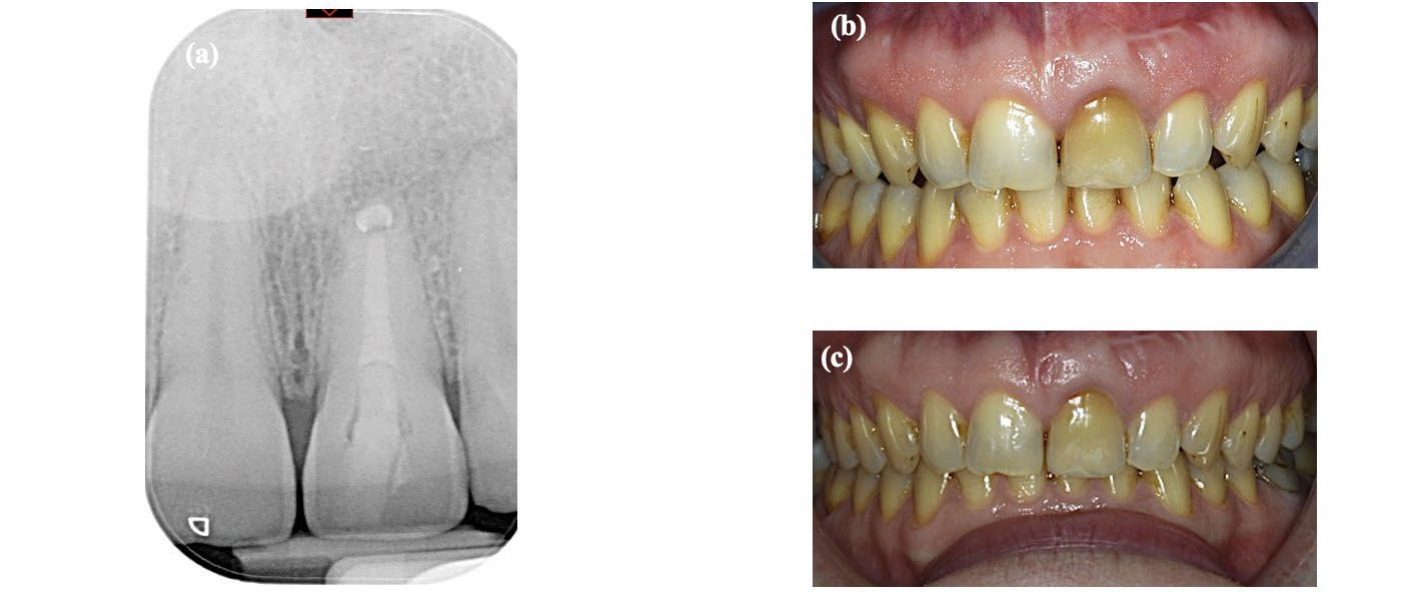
a) A periapical radiograph of a root canal-treated left maxillary central incisor. b) A pre-operative photo showing an endodontically treated tooth discolouration. The tooth was classified as C4 using the VITA Classical Shade Guide. c) The tooth was managed using inside-outside bleaching using 10-15% carbamide peroxide in a bleaching tray; however, the discolouration was still present and the tooth was indicated for veneers
